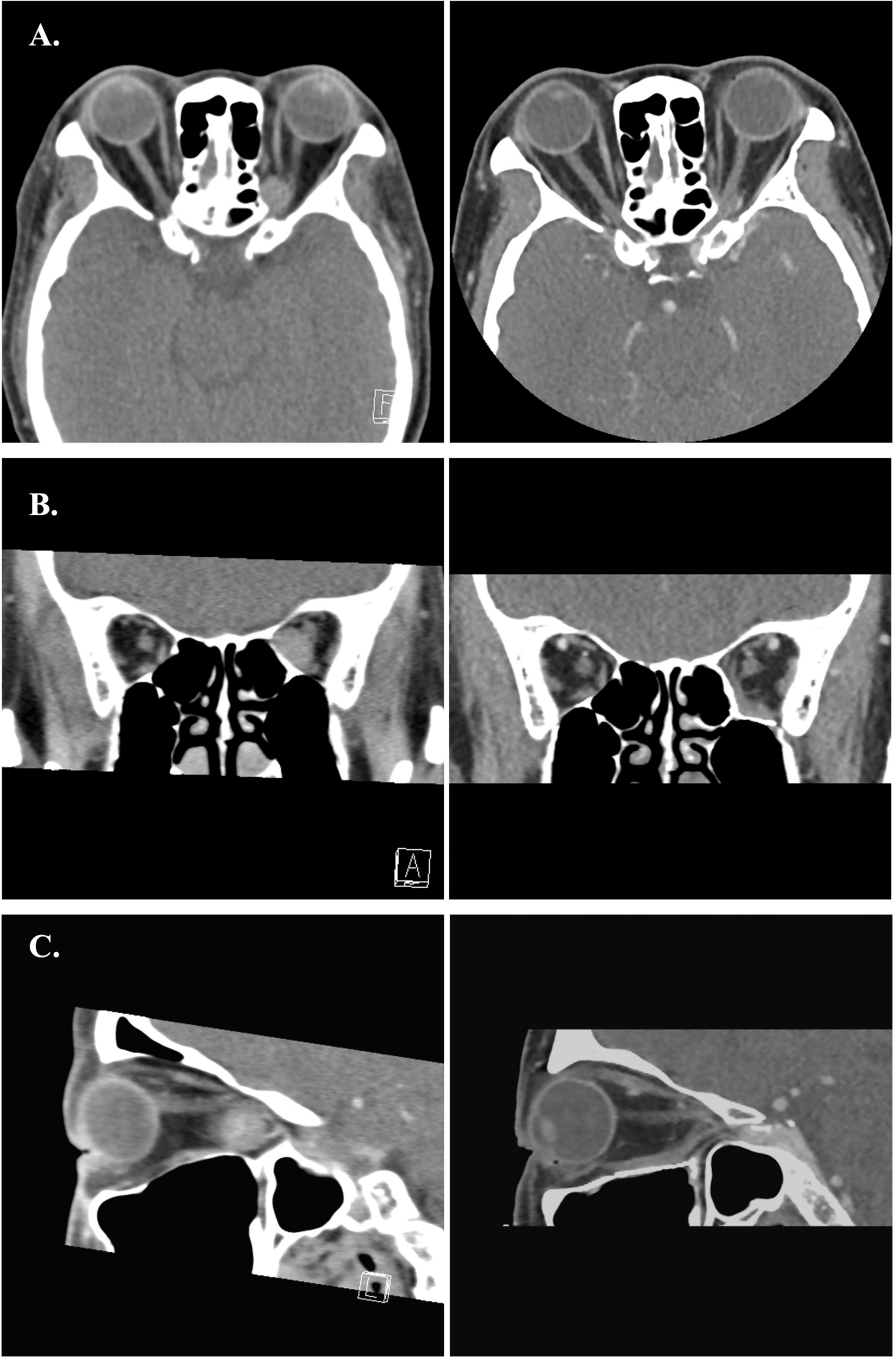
(A–C) Preoperative and postoperative images of Case 2. The left-side axial, coronal and sagittal images were the preoperative CT scans. The right-side postoperative CT images showed normal extraocular muscles position and well restored orbital volume with Titanium mesh.
